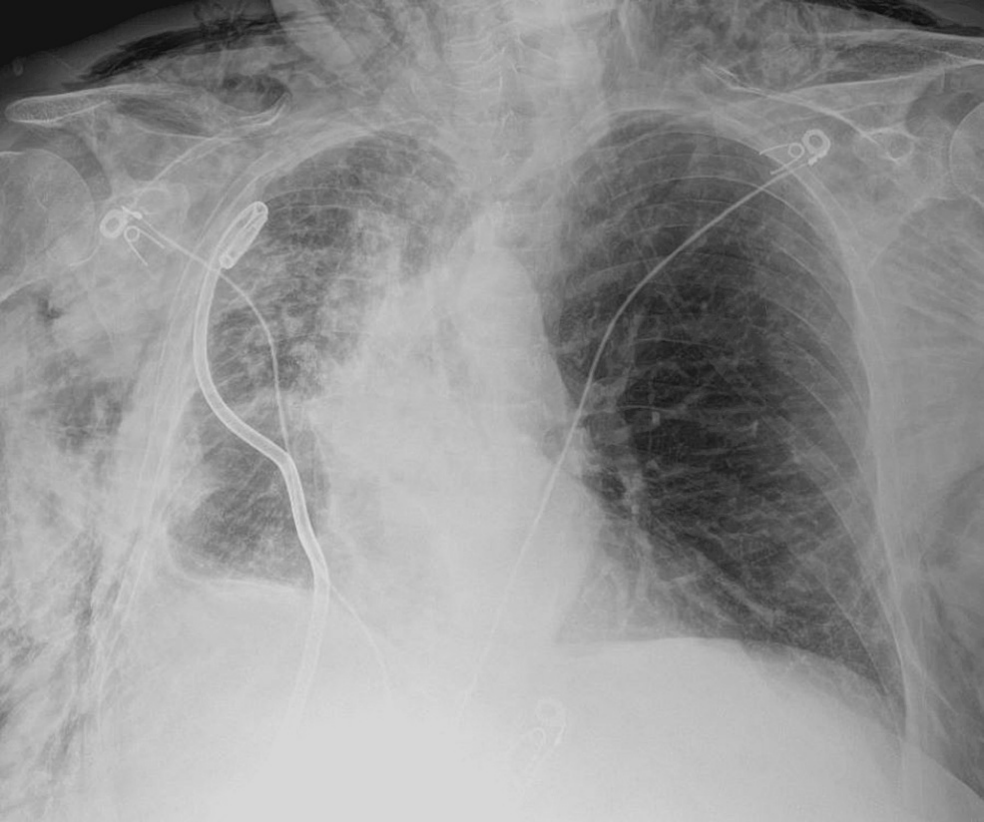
Chest radiograph following explantation of the RML valve resulting in significant lung re-expansion and resolution of continuous air leak RML:
